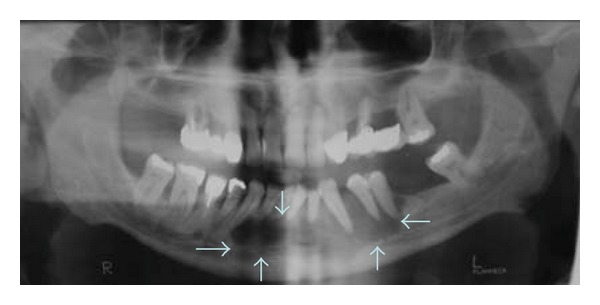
Case 1. Panoramic radiograph showing lytic multilocular lesion: mandible.
